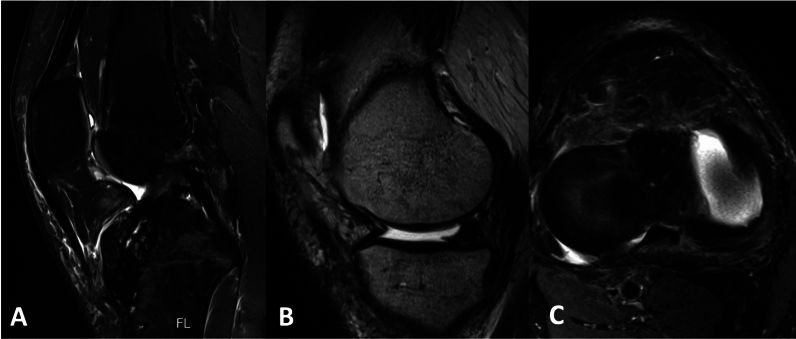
Example of a clinical case of symptomatic meniscal tear. A 37-year-old patient, 8 months after ACL and anterolateral ligament reconstruction with medial meniscus repair, presented with new-onset pain without a new traumatic event. Stability tests were negative. MRI demonstrated: A. Properly positioned tunnels with graft in the healing phase and a double-PCL sign; B. Medial meniscus tear on T1 sagittal sequence; C. Bucket-handle tear on axial Proton Density Fat-Suppressed (PD-FS)
